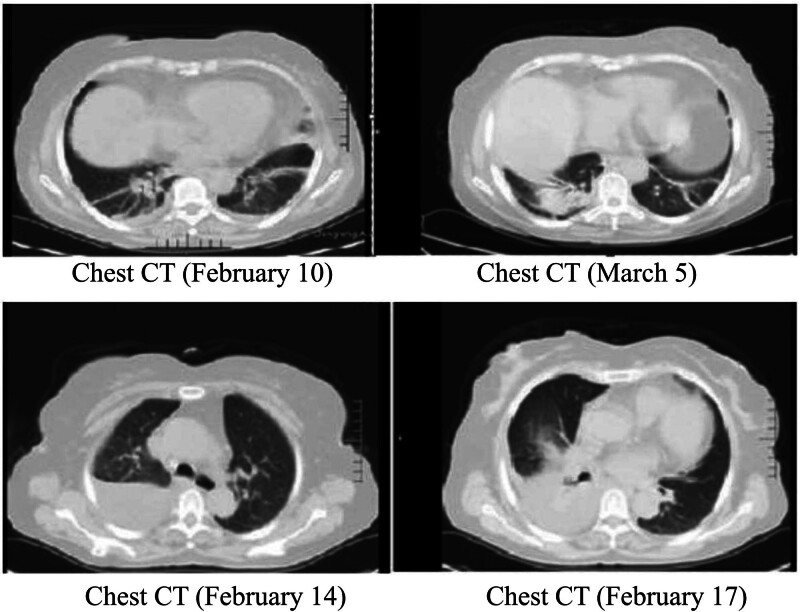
Chest CT. CT = computed tomography.
